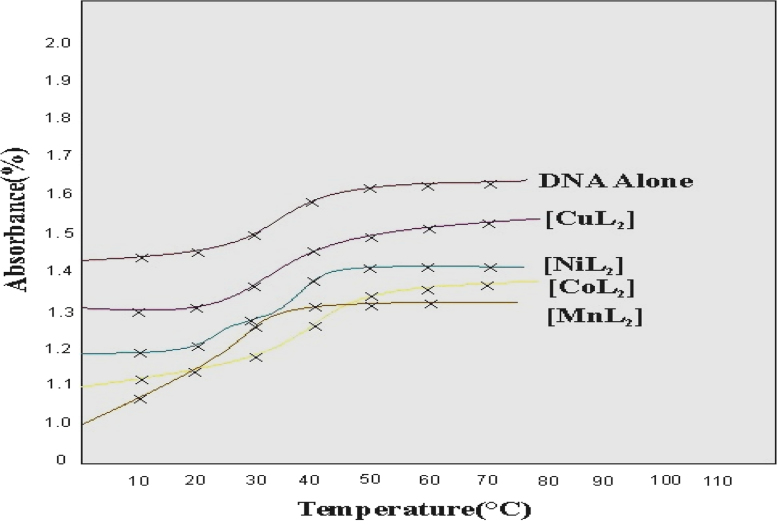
Melting curves of CT-DNA in the absence and presence of copper complex.
